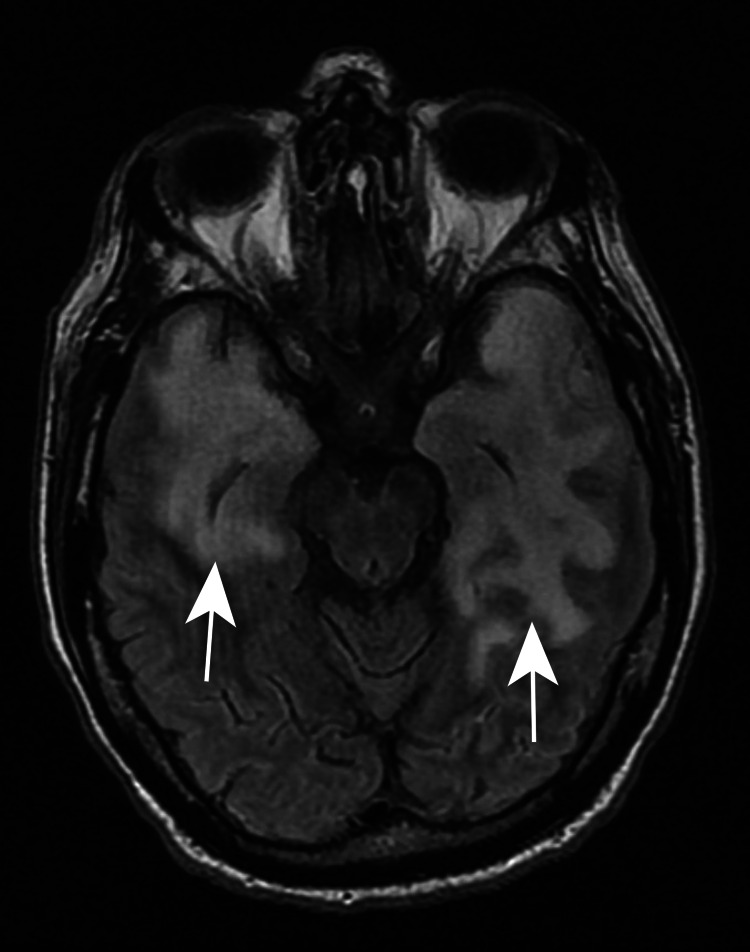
MRI of the head with extensive confluent vasogenic edema within the bitemporal lobes
